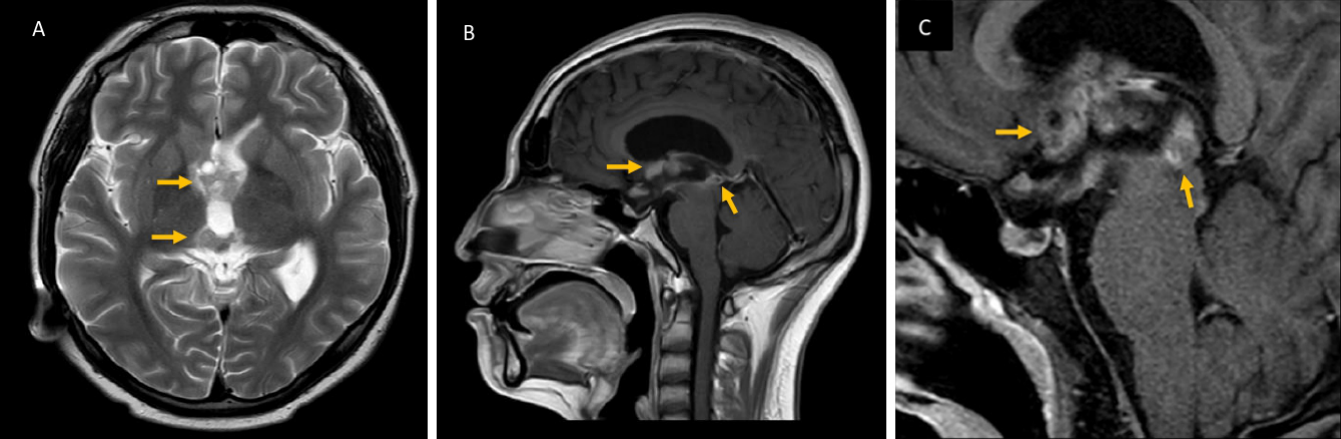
Brain magnetic resonance imaging (MRI) findings. A. T2WI MRI Axial Lesions common isointense and hyperintense in the third ventricle with multiple cysts in the germinoma. B. Sagittal T1WI C+ MRI shows homogeneous suprasellar enhancement of the hypothalamus and chiasmatic region, with extension to the pineal gland through the floor of the third ventricle. C. Sagital T1WI C MR. Large suprasellar lesion with obstructive hydrocephalus with the appearance of an enlarged lateral ventricle and flattening of the fornix.
